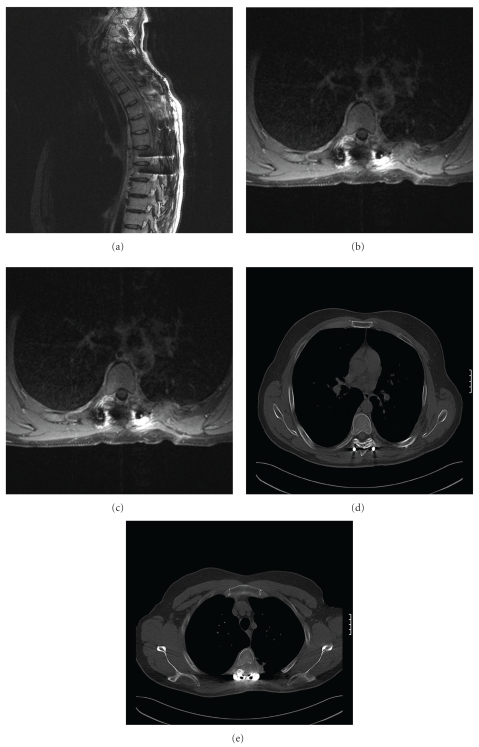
Postoperative MRI (a, b, c) and CT scan (d, e) show no evidence of recurrence six months after the operation.
